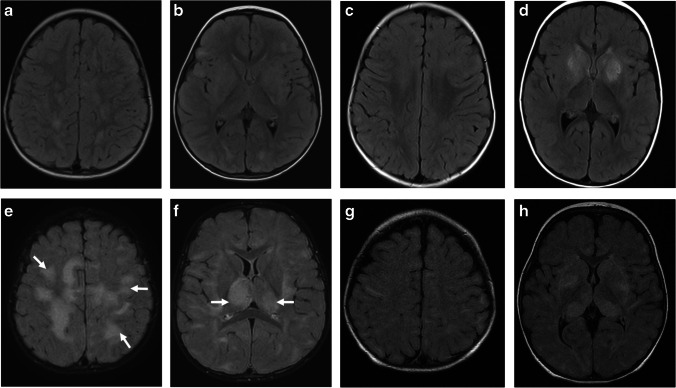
Acute disseminated encephalomyelitis (ADEM) pattern: axial fluid-attenuated inversion recovery (FLAIR) magnetic resonance images of the brain obtained from a 29-month-old boy (a, b), a 20-month-old girl (c, d), a 19-month-old boy (e, f), and a 15-month-old boy (g, h) with myelin oligodendrocyte glycoprotein antibody seropositivity, who presented with ADEM. Images at the level of the fronto-parietal lobes (a, c, e, g) and deep gray matter nuclei (b, d, f, h), show varying appearances of involvement of white matter (*arrows* in e) and deep gray matter structures (*arrows* in f)
